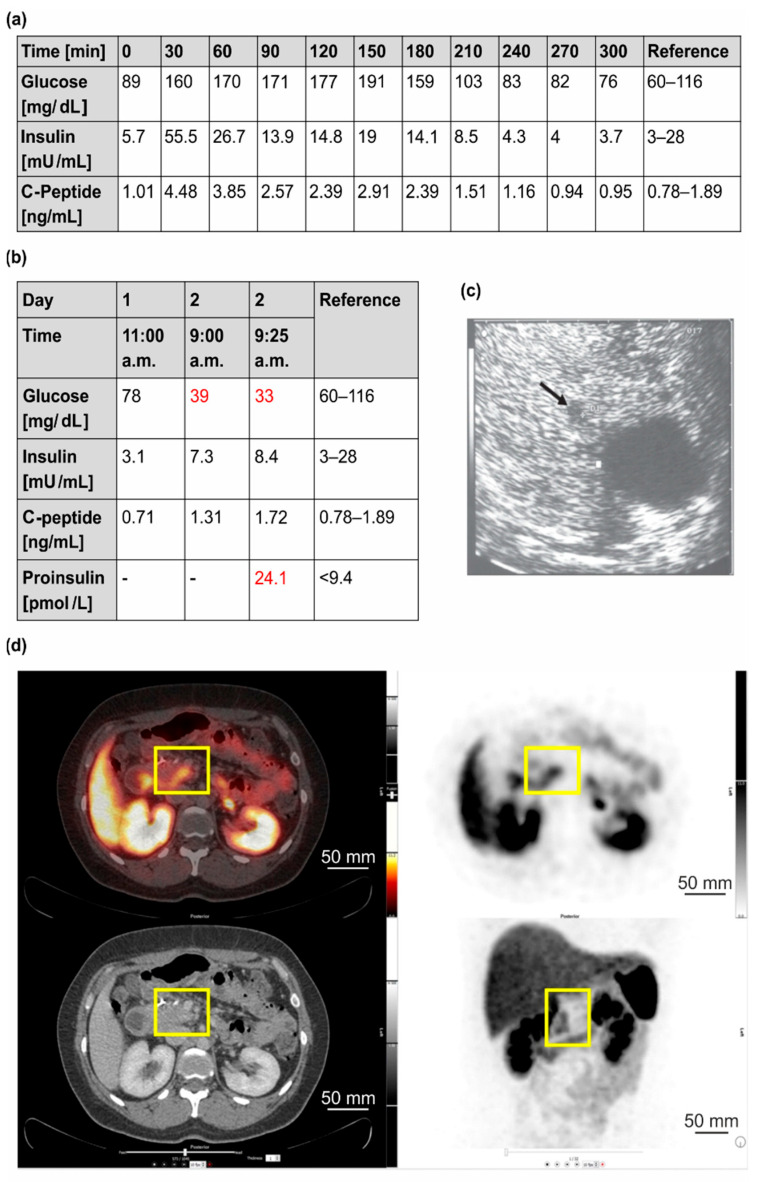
Laboratory tests and diagnostic imaging of case 1 (index patient, IV4). (a) Endocrine testing showed unremarkable oral glucose tolerance (prolonged test conditions) without clinical or biochemical signs of hypoglycemia. (b) A 72 h fasting test showed marked hypoglycemia after 22 h with a plasma glucose as low as 33 mg/dL and corresponding serum C-peptide, insulin, and proinsulin levels inadequately elevated. (- = not performed). Values outside the reference range are highlighted in red. (c) Further imaging with endoscopic ultrasound revealed a single lesion of 4–5 mm. (d) Functional imaging with 68Ga-DOTATOC-PET/CT was negative with low and diffuse uptake in the pancreatic head (yellow frames), interpreted as unspecific.
